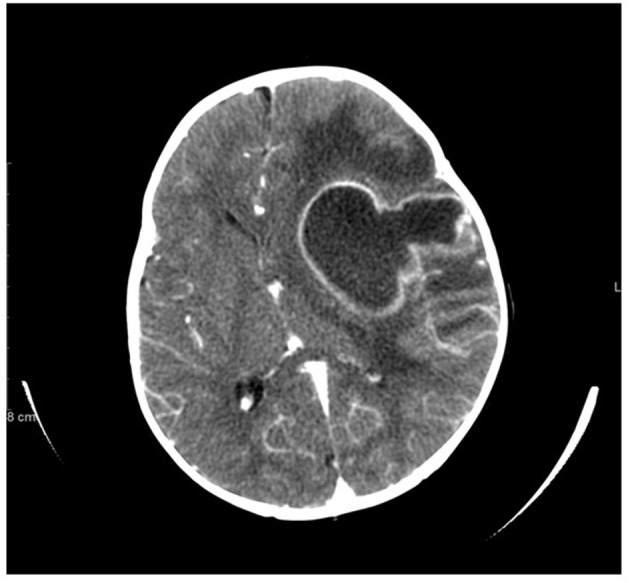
Non-contrast CT scan of brain at presentation demonstrates large left cavitary abscess with inflammatory sediment, left hemispheric edema, ventricular compression, severe mass effect, and midline shift.
